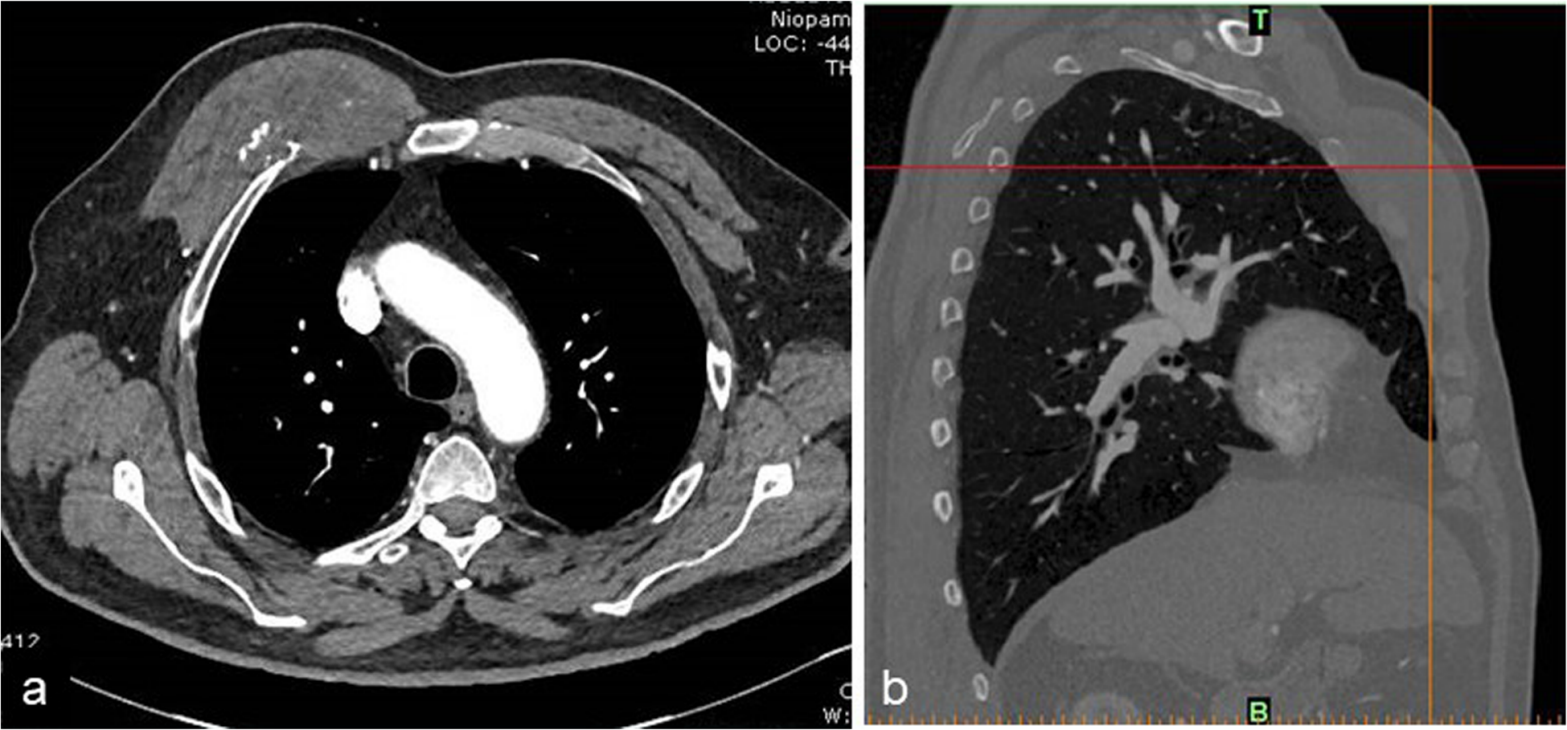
CT scan of the chest. Axial view (a). The tumour is located deep to the right pectoralis major muscle with scalloping of the anterior surface of the third rib. The sagittal view shows the tumour lying over the 2nd, 3rd and 4th ribs anteriorly (b)
